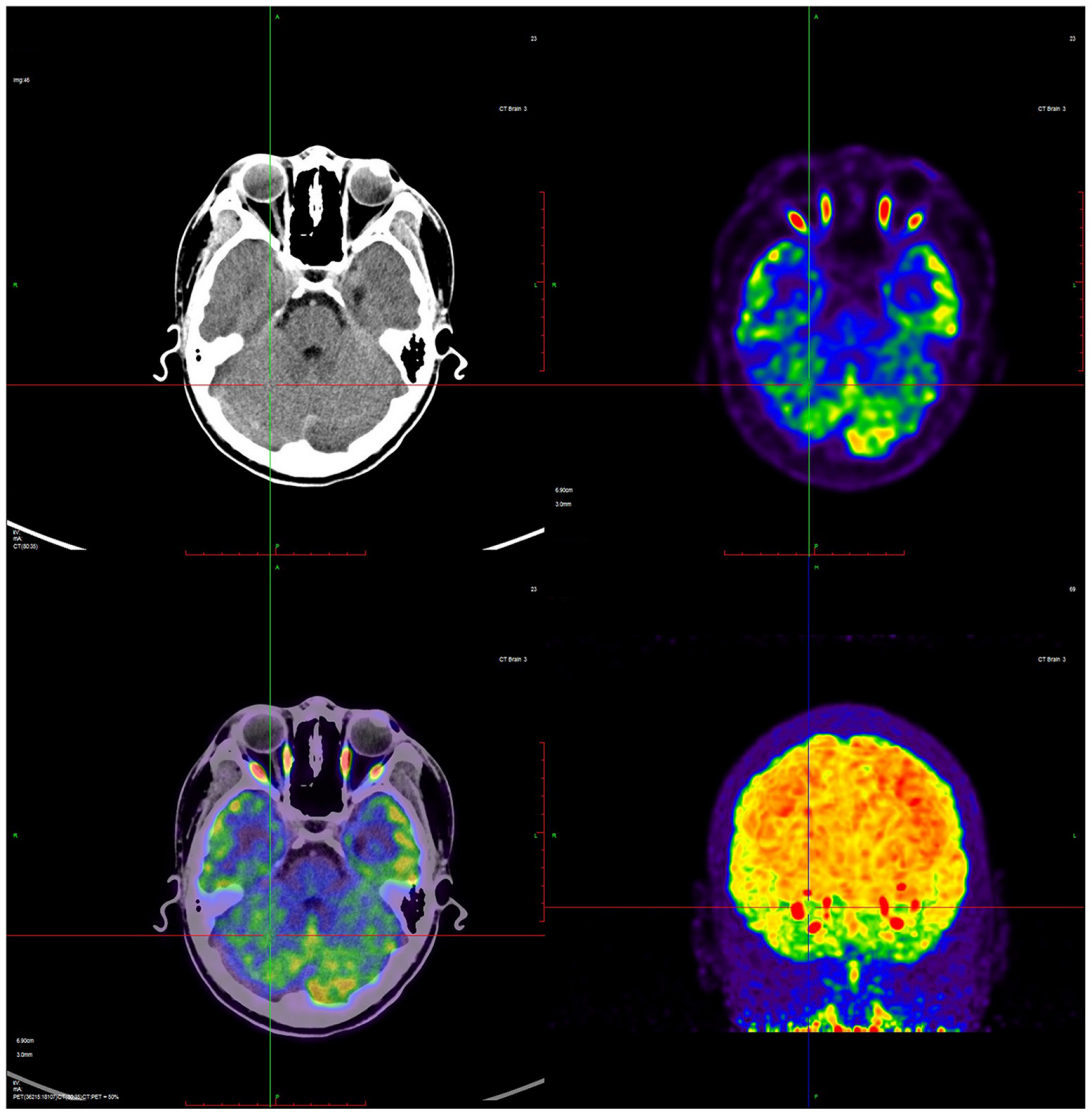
A representative ^18^F-FDG brain PET image from a CP child showed the relative higher glucose metabolism in left cortex. Red color indicates relatively high glucose metabolism and blue relatively low in comparison to corresponding regions.
